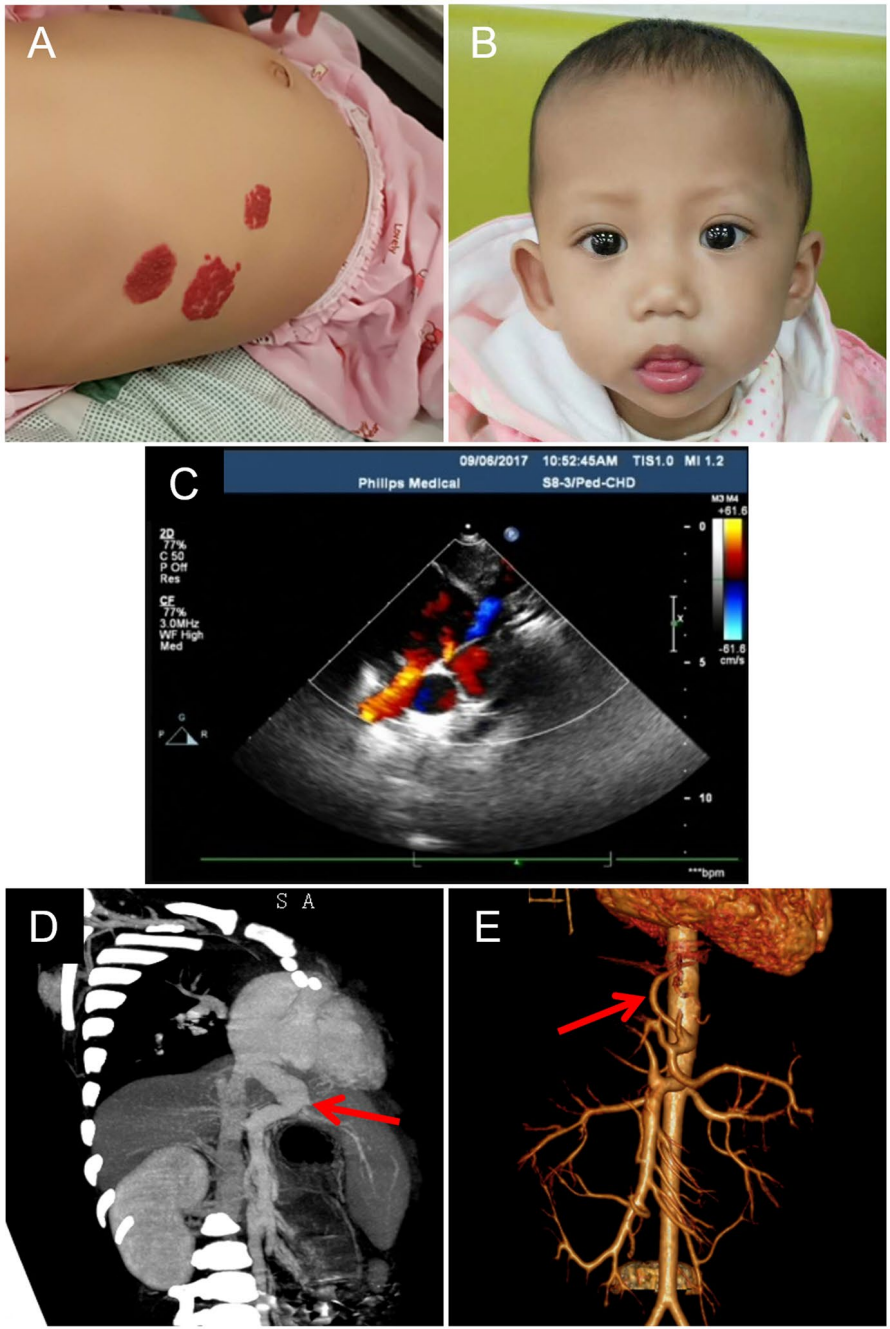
Skin capillary malformations, facial feature, and heart and liver arteriovenous malformations of the patient. (A) Multiple flaky purplish red hemangiomas presented in the abdomen. (B) The characterized face of the patient shows a broad forehead, a small-pointed chin, and prominent ears. (C) The image of color Doppler b mode ultrasonography shows congenital heart disease: patent ductus arteriosus, patent foramen ovale, tricuspid, and mitral regurgitation. (D) The CT image shows enlarged portal vein draining directly into the inferior vena cava (red arrow). (E) Angiography shows enlarged left hepatic artery (red arrow).
